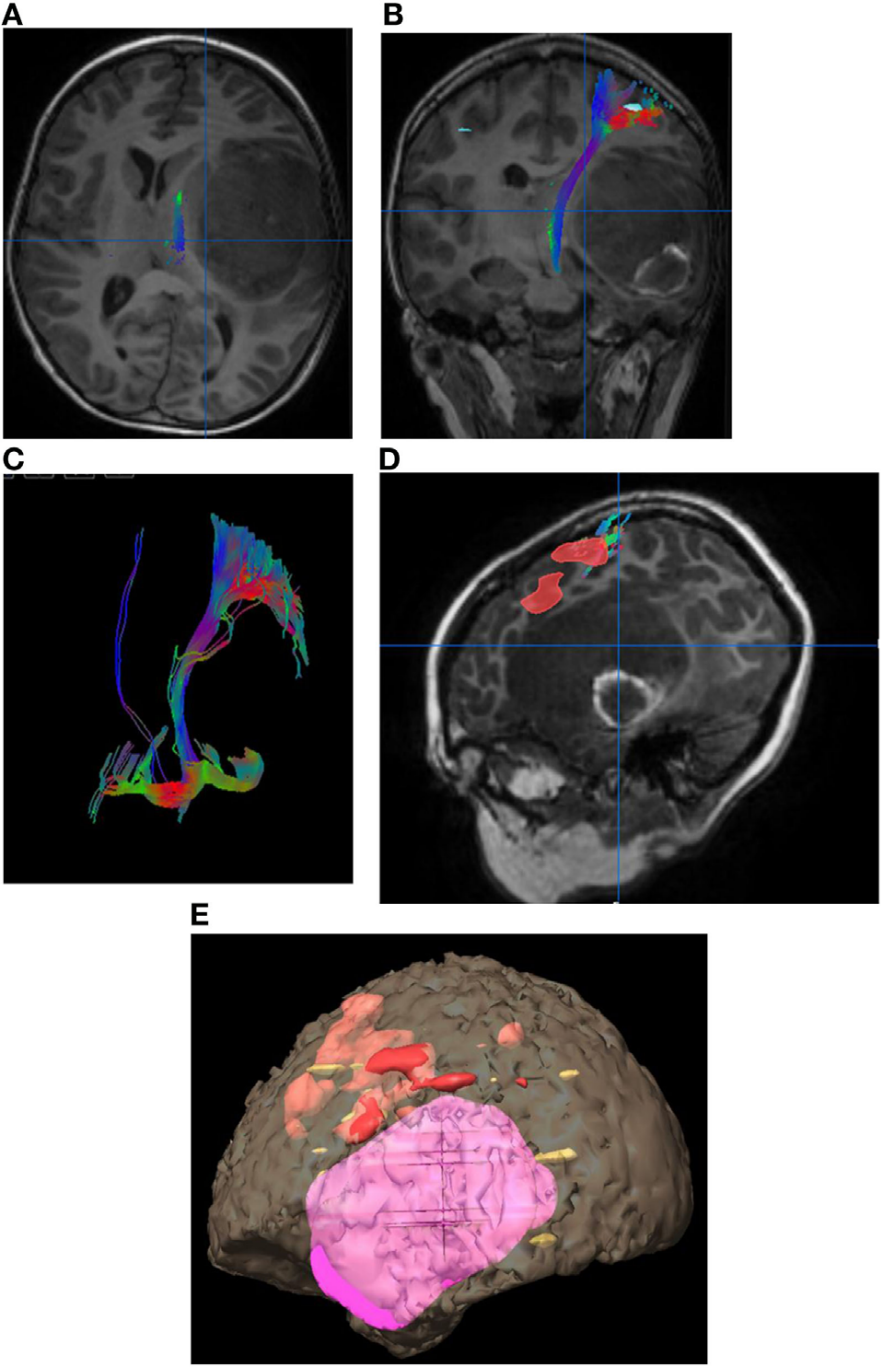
Eight-year-old right-handed boy with left temporal lobe glioblastoma. (A) Tractography in the axial plane demonstrating the left corticospinal tract (CST). (B) Tractography of the left CST in the coronal plane. (C) Three-dimensional fiber tracking of the left CST. (D) Sagittal T1-weighted image with fused language activation colored red demarcating Broca’s area, which is elevated by the left temporal lobe tumor. (E) Three-dimensional reconstruction with the tumor in pink and task-based functional areas of eloquent language in red, yellow, and orange. No copyright permissions were required to use these images.
